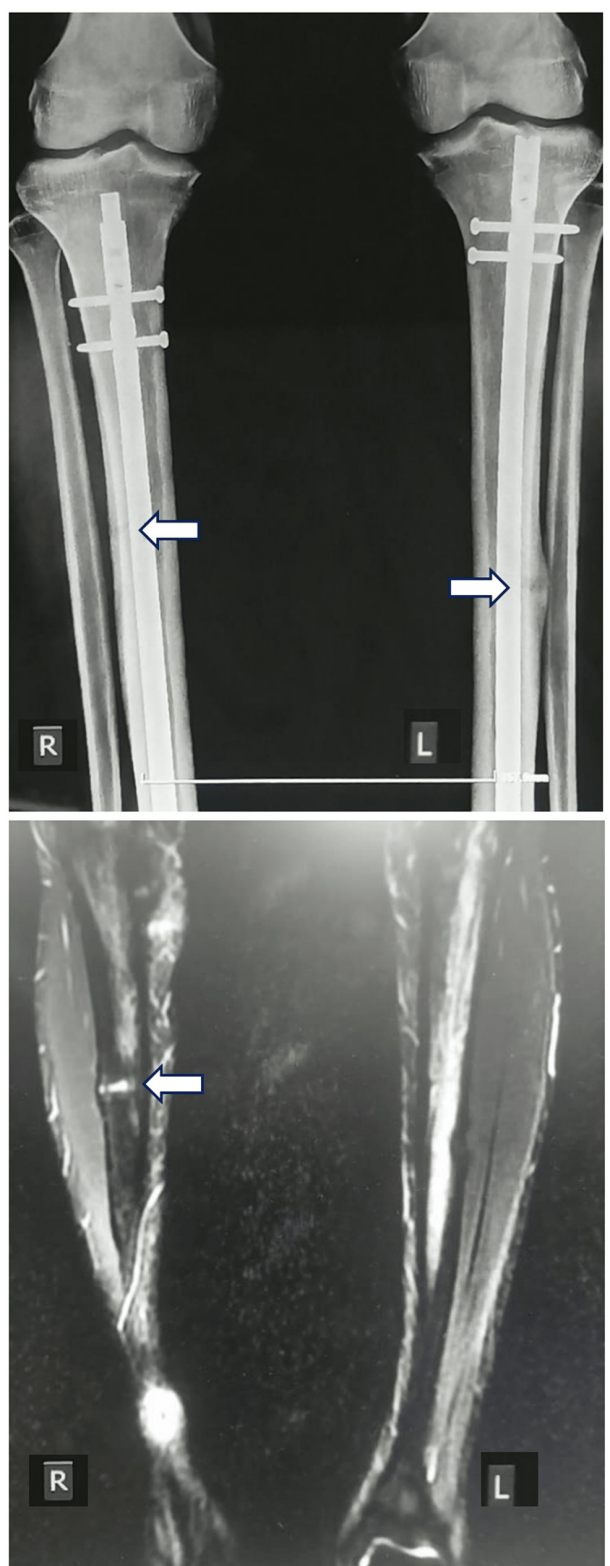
Primary surgical treatment with IMN and evidence of nonunion Top: plain radiographs for both tibias 10 months after the placement of intramedullary nails; right tibia: dreaded black line indicating nonunion; left tibia: bone healing with the formation of hypertrophic mature callus. Bottom: fat-suppressed coronal T1-weighted MRI scan revealing a persistent fracture line (arrow) at the midshaft of the right tibia IMN: intramedullary nailing; MRI: magnetic resonance imaging
